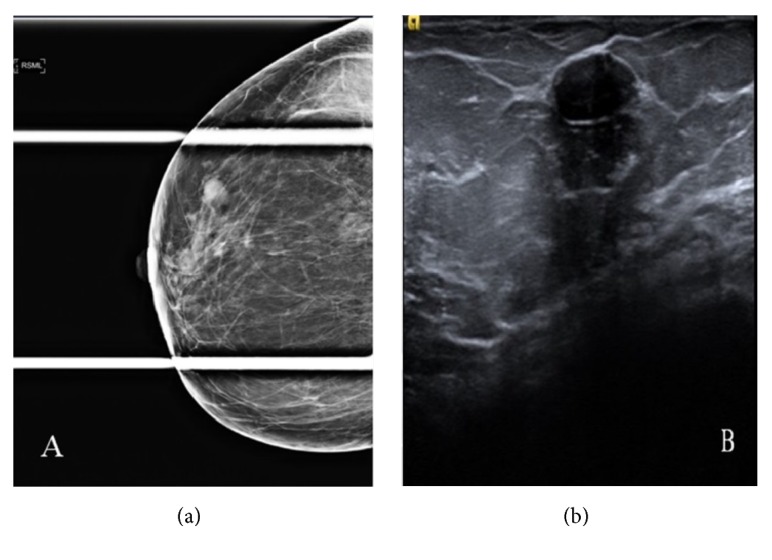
53-year-old woman presenting for evaluation of a palpable mass. (a) Spot compression view at the site of the palpable abnormality shows an oval circumscribed equal density mass. (b) Ultrasound confirms an oval circumscribed hypoechoic mass with parallel orientation. A biopsy was recommended in view of the new palpable finding. The mass remained stable at follow-up after 18 months.
